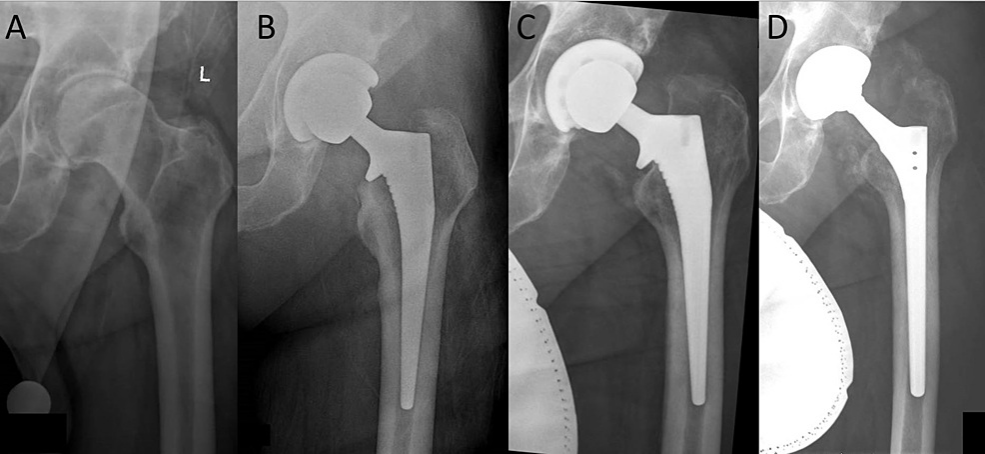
Radiographs of patient 2. (A) Preoperative AP of patient 2 with Dorr A bone and canal-flare index of 7.8. (B) Postoperative AP of Corail size 11, collared, coxa vara stem with a +5 mm head. (C) Follow-up radiographs with varus migration. (D) Revision with Wagner monoblock stem. AP, anteroposterior view
